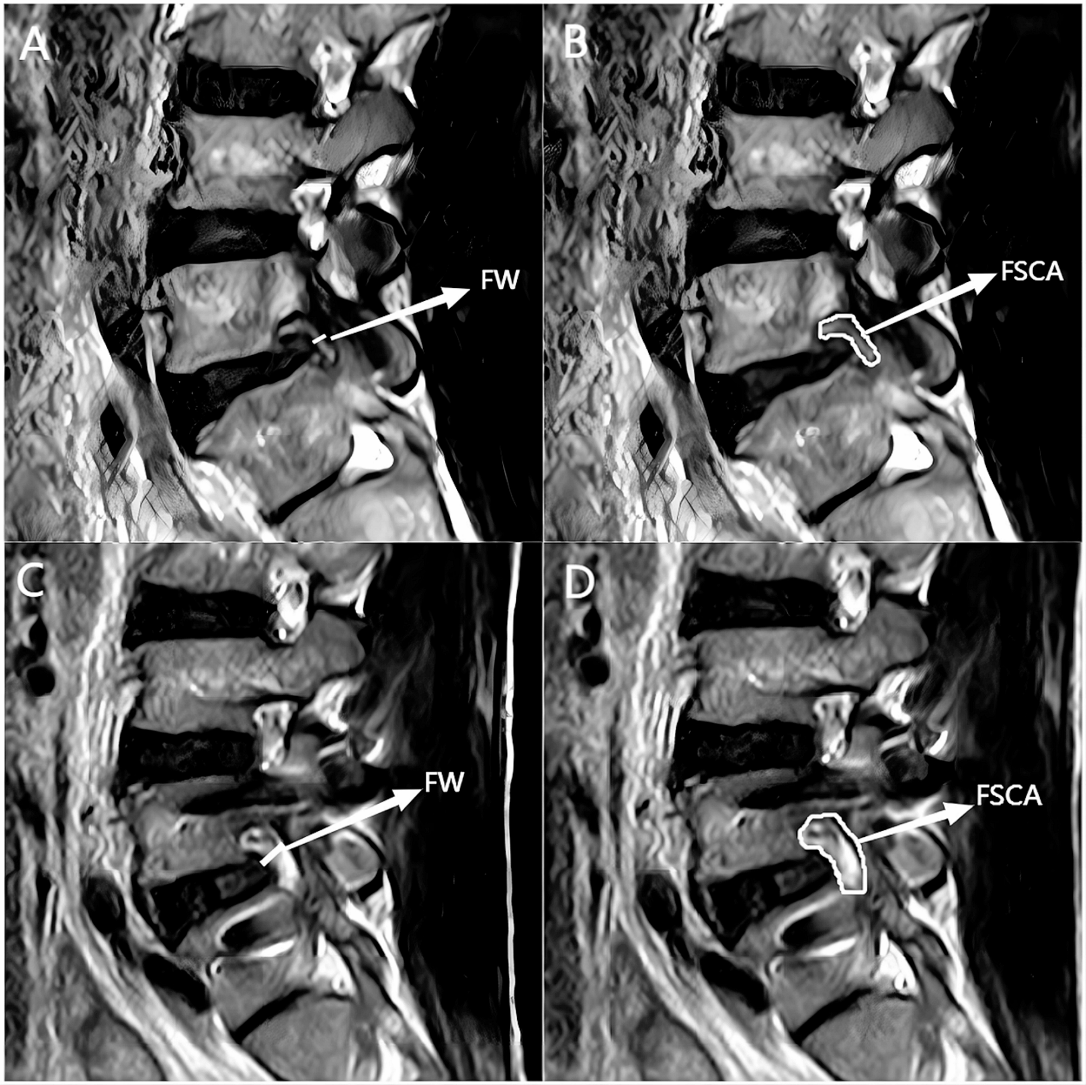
The foraminal cross-sectional area (FSCA) in the middle area of nerve foramen in T2-weighted MRI sagittal position was measured. The foramen width (FW) was the distance between the intervertebral disc in the middle region of the nerve foramen and the narrowest point of the posterior ligamentum flavum measured by T2-weighted MRI in sagittal position of the lumbar vertebra. A and C are the width of intervertebral foramen before and after operation, and B and D are the area of intervertebral foramen before and after operation
